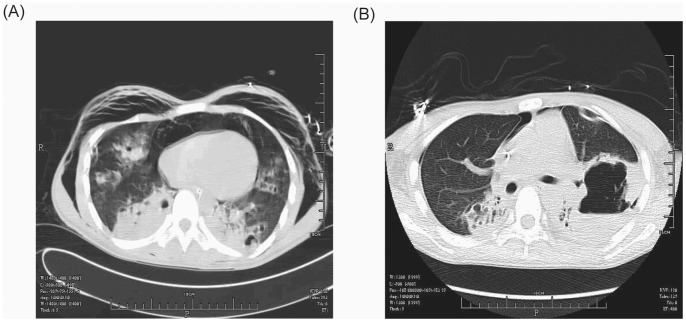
Lung CT scan on (A) day 7 of hospitalization showing abscess formations and (B) day 26 of hospitalization showing a large abscess cavity with a fluid level in the left lobe.
